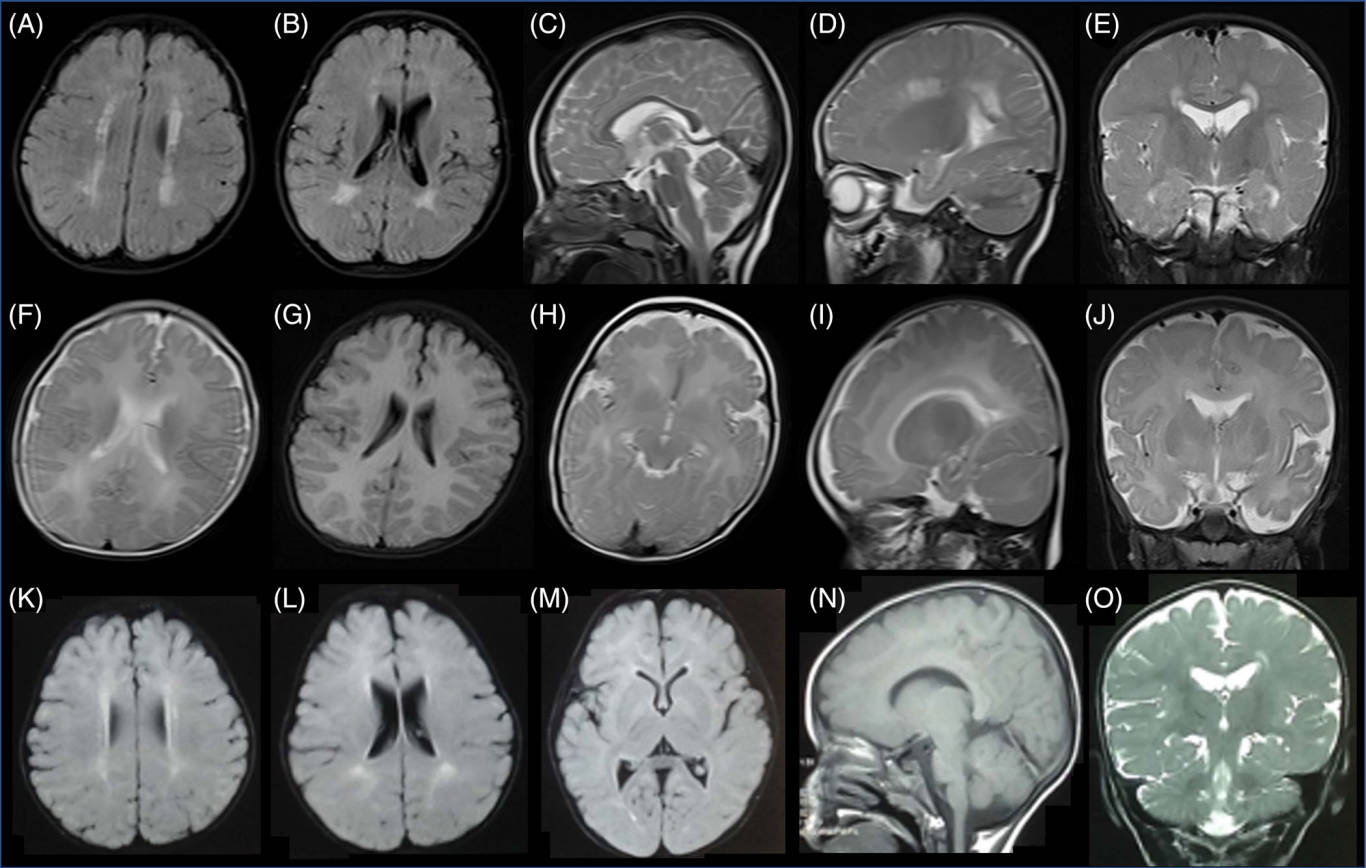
Brain MRI findings of patients with *DPM3* variants. Findings of patient 1 (A–E) include T2/FLAIR hyperintense foci in the subcortical and periventricular white matter (A, B, D, E), mildly prominent ventricles secondary to white matter volume loss (B, C, E), and corpus callosum hypoplasia (C). Findings of patient 2 (F–J) include hypomyelination, T2 hyperintense foci in the periventricular white matter (F, H, I, J), mildly prominent lateral ventricles (G, J), and slightly prominent frontal and temporal subarachnoid spaces (H, J). Findings of patient 5 (K‐O) include T2/FLAIR hyperintense foci in the subcortical and periventricular white matter (K, L) and globi pallidi (M), mildly prominent lateral ventricles, white matter volume loss (L, O), and mega cisterna magna (N). [Colour figure can be viewed at wileyonlinelibrary.com]
